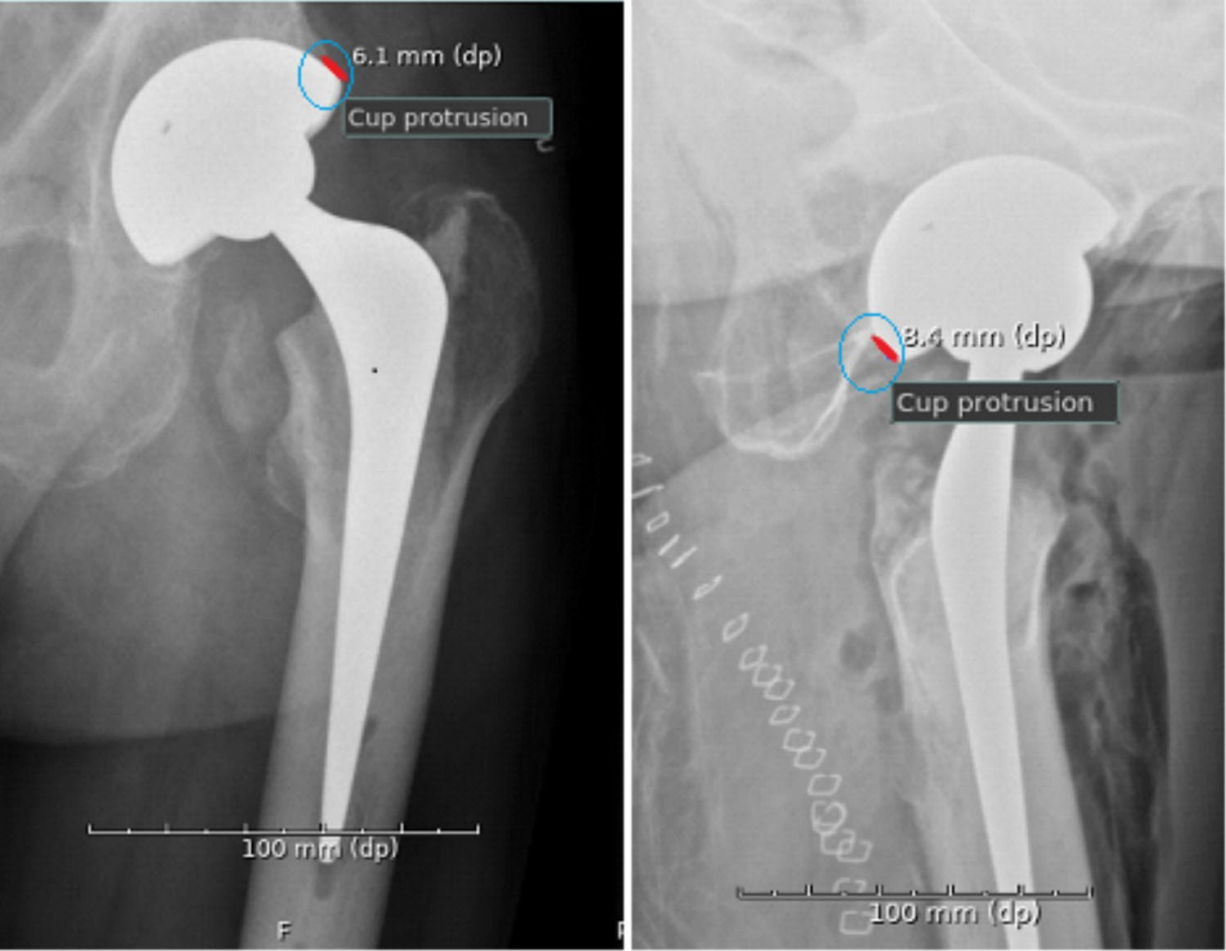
Cup protrusion measured on AP pelvic radiograph and a cross-table lateral radiographs
